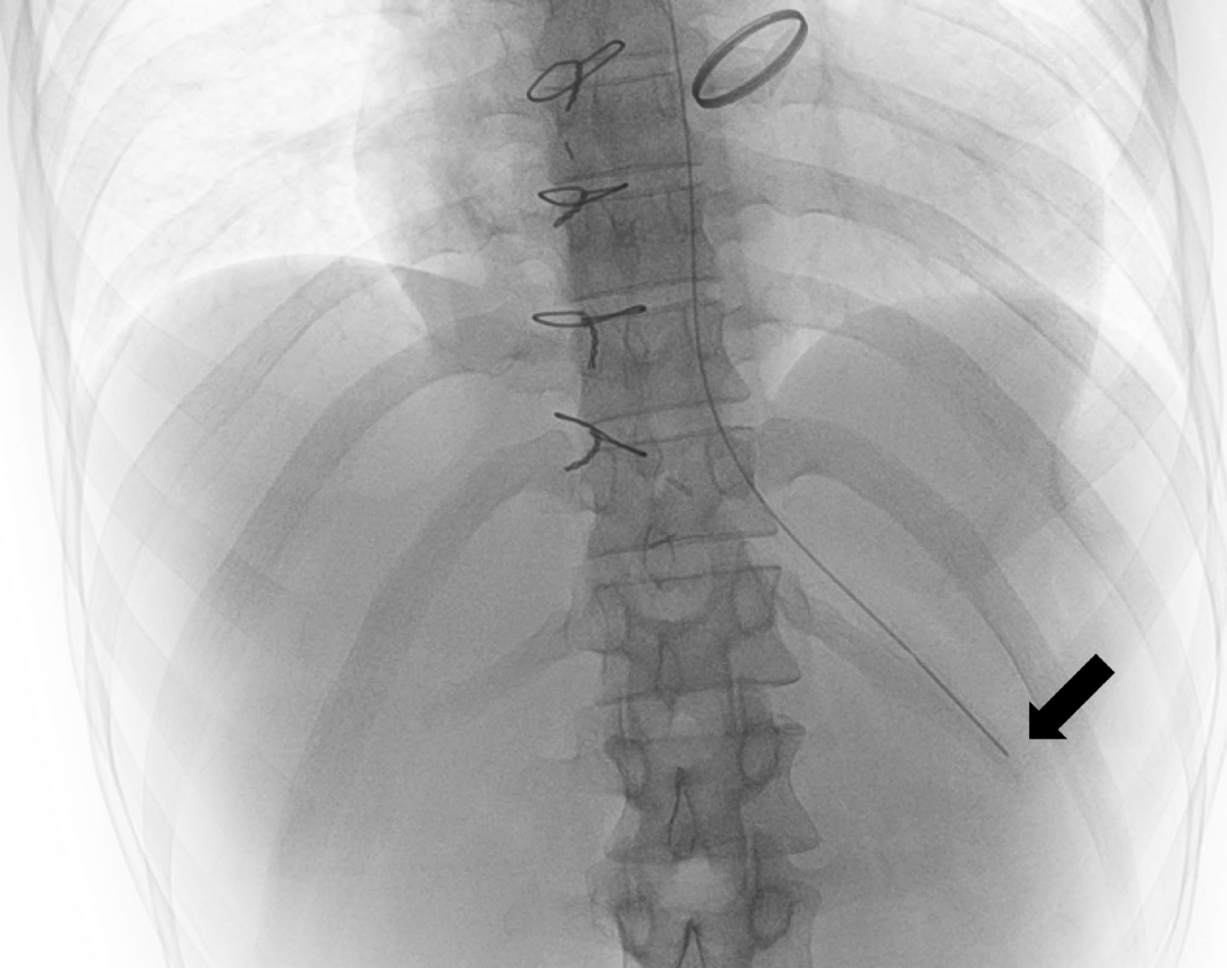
Chest radiograph demonstrating correct intragastric positioning of the nasogastric tube. The tube tip is located within the stomach (arrow), confirming appropriate placement prior to the initiation of enteral nutrition
